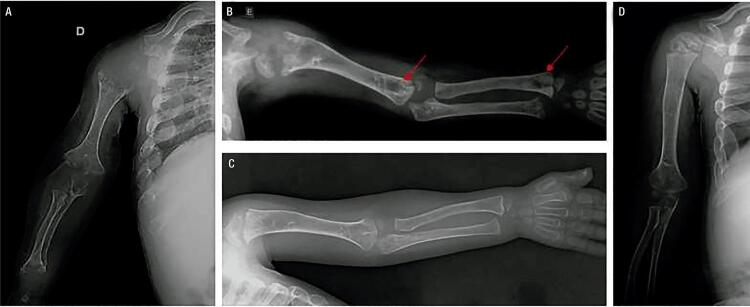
Upper limb radiographs. (A) Age 1 year and 2 months: diffuse bone hypomineralization, cupping, fraying and widening metaphyses, thin cortical bone, and diaphyseal shortening. (B) Age 2 years and 10 months, prior to treatment: metaphyseal “tongues” of radiolucency (arrows). (C) Age 4 years, after 1 year of treatment. (D) Age 5 years, after 2 years of treatment: improvement of the signs of rickets. Treatment improved bone mineralization and formation, cupping, fraying and widening metaphyses, and radiolucent “tongues”. The long bone became better defined, and the lytic and sclerotic metaphyseal areas resolved.
